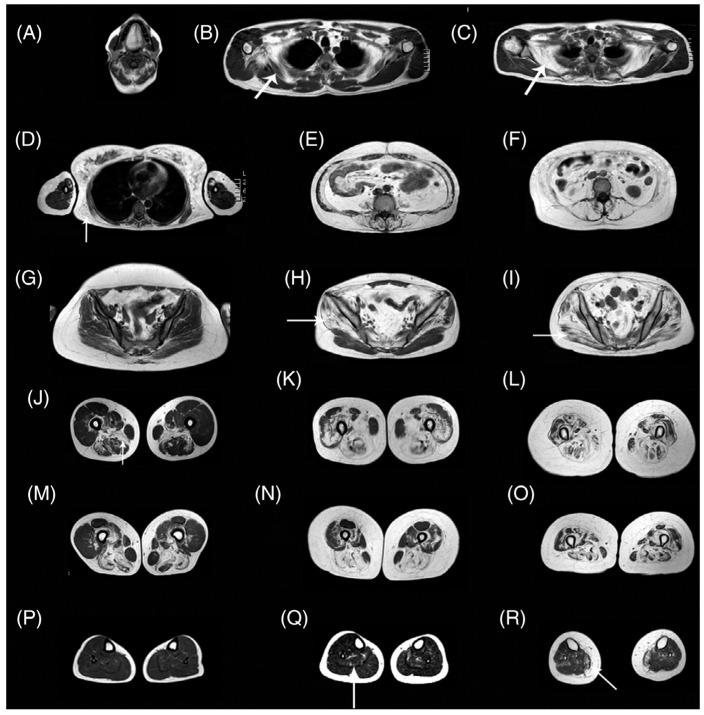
Whole-body T1w imaging of patients with late-onset Pompe disease. (A) Involvement of tongue is observed. In the scapular girdle, the subscapularis (arrow in B,C) and latissimus dorsi (arrow in D) are affected, yet the deltoid, biceps, and triceps are not typically involved. Paraspinal and abdominal muscles are typically affected (E,F). The gluteus minimus and medius (arrow in H) are affected earlier than the gluteus maximus (arrow in I). Patients in the early stages of disease may have no glutei involvement (G). In the thigh, the adductor magnus and long head of biceps are involved earlier (J,M), whereas posterior muscles and the vasti are affected later in the progression (K,N). Eventually, all muscles of the thigh are affected (L,O). A proximal-to-distal gradient in the vasti is usually identified (J–M,K–N), although it is lost in advanced stages (L–O). Lower legs are usually spared (P), although mild replacement of the soleus (arrow in Q) and media gastrocnemius (arrow in R) can be observed. The images shown are from seven patients. T1w, T1-weighted. Reproduced with permission from the authors in Ref. [115].
